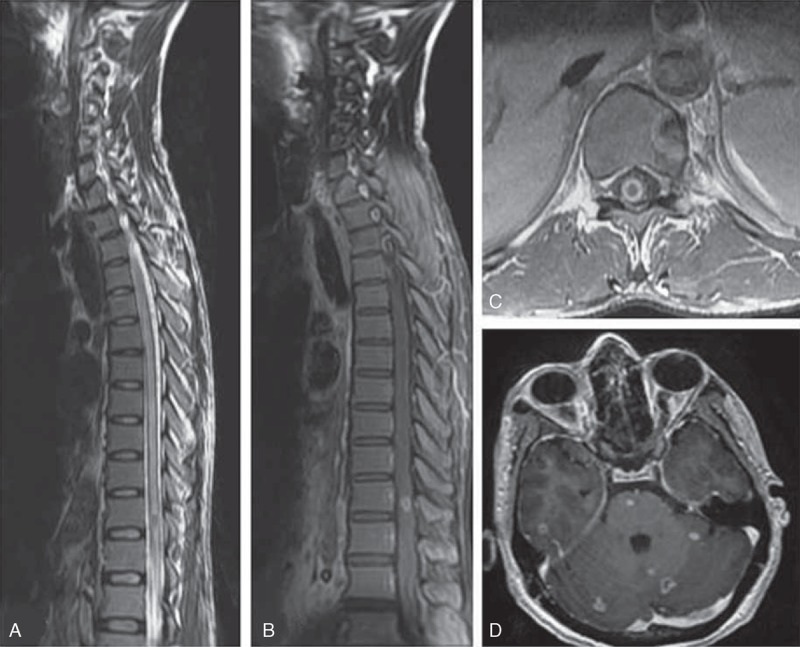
MRI spine of a 55-year-old man with tuberculous meningitis, who presented with a 3 weeks history of paraparesis, MRI showed long segment myelitis (A) and intramedullary tuberculoma at D10 level (B and C). MRI brain of the patient showed multiple tuberculoma involving cerebrum, cerebellum, and brainstem (D). MRI = magnetic resonance imaging.
